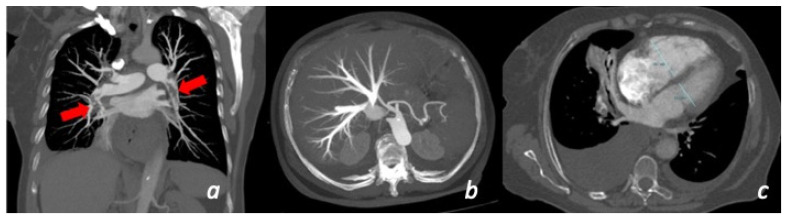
Patient with chest pain and dyspnea for more than 10 days with recent worsening of symptoms. CT shows the presence of massive bilateral pulmonary thrombo-embolism (red arrows) in the right and left lower lobe pulmonary artery (a). Secondary overload of the right-side circulation: enlargement of the right ventricle (c) and secondary tricuspid insufficiency (b).
